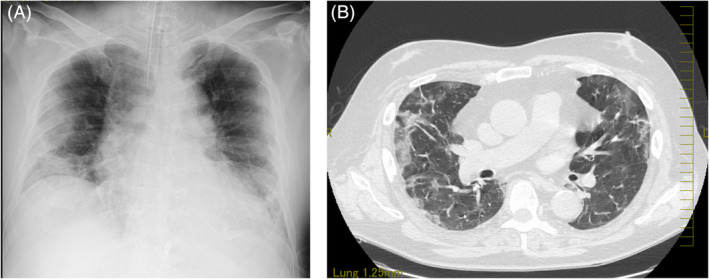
(A, B) X‐ray and computed tomography (CT) of the chest on admission demonstrated diffuse ground‐glass opacity located predominantly at the pulmonary periphery.
